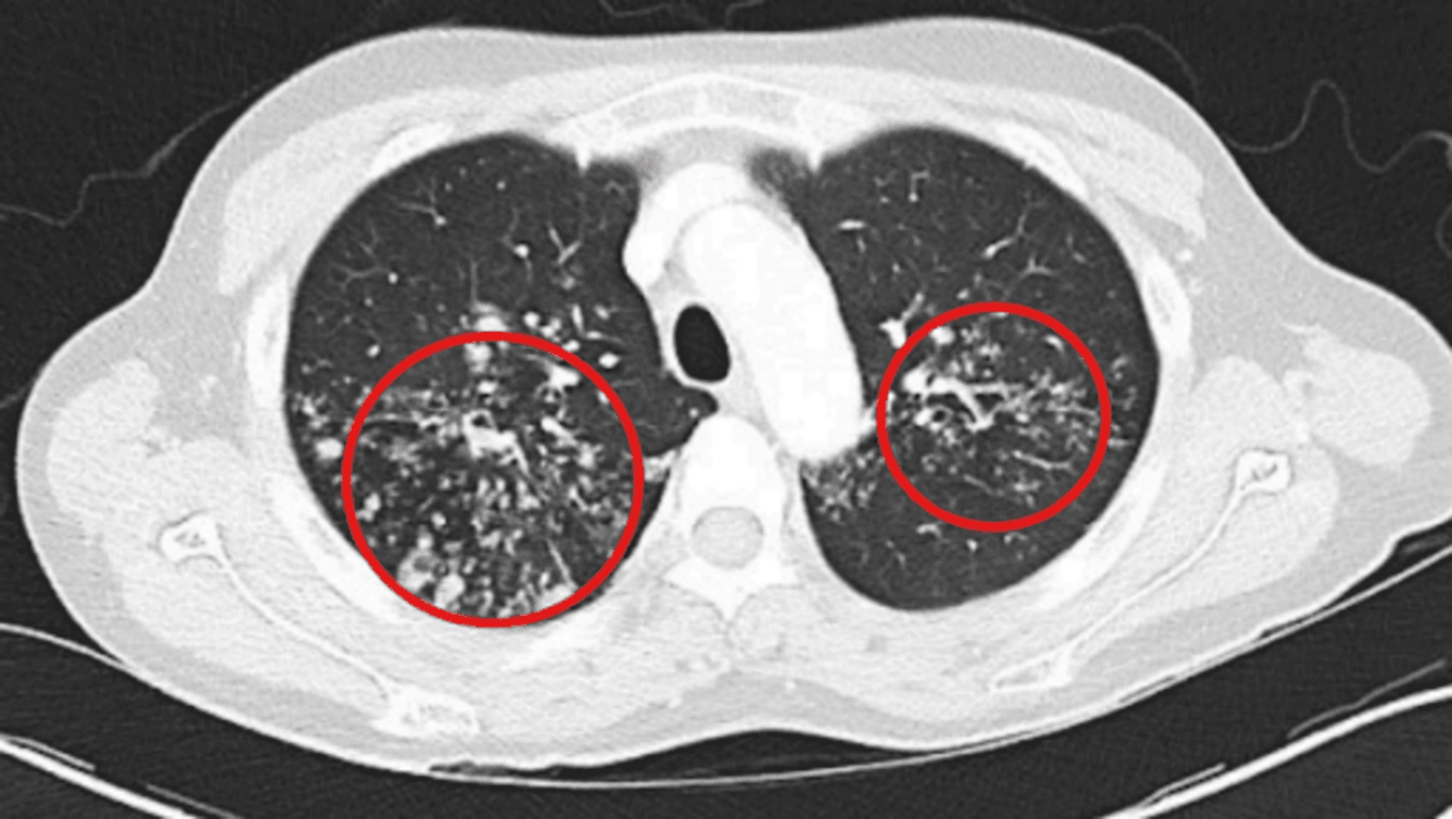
Computed tomography of the chest demonstrating innumerable solid pulmonary lesions with multiple large cavitary areas predominantly involving the upper lobes
